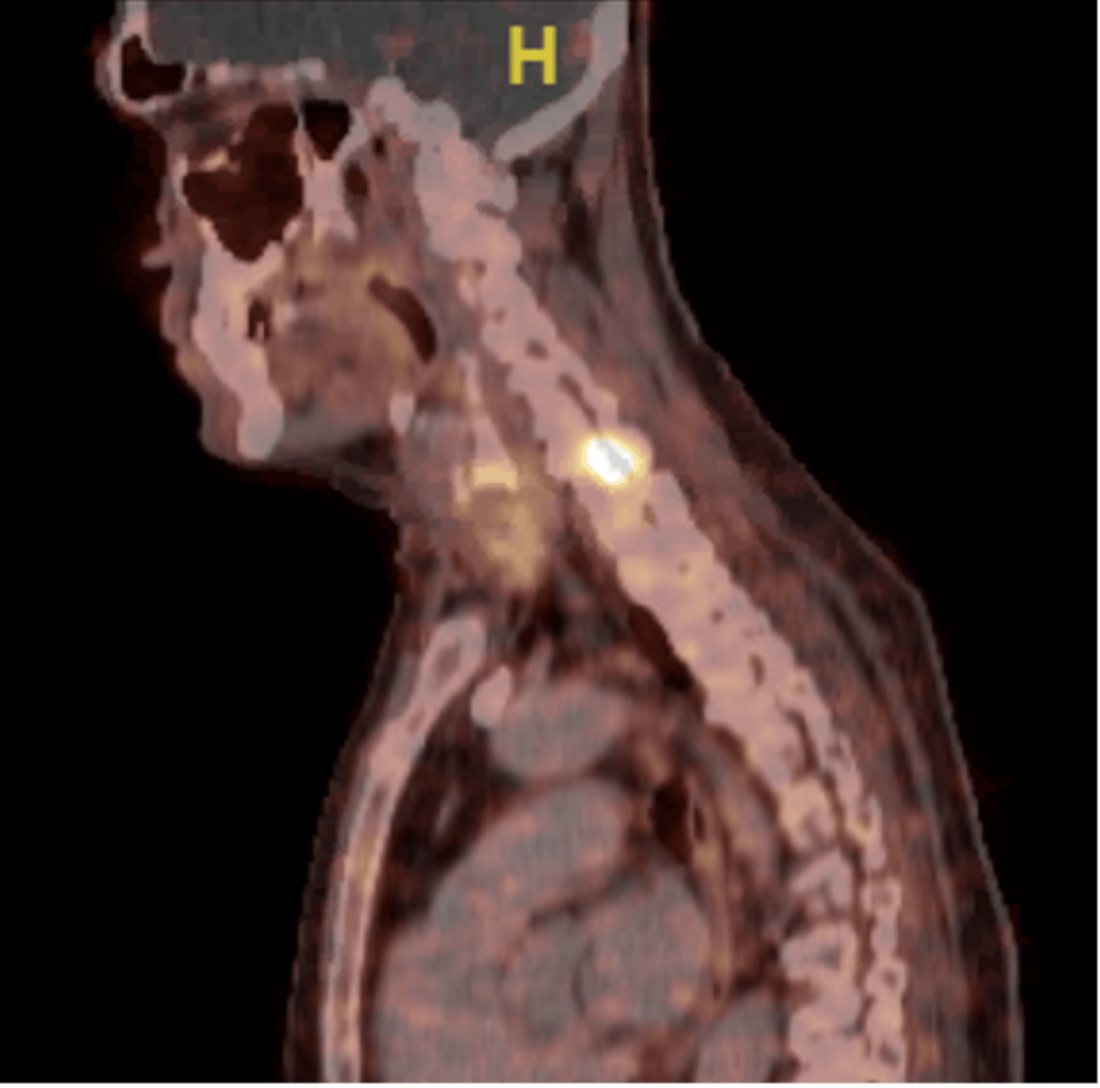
Hyperdense lesion in C7-T1 concerning for a mesenchymal tumor Radiographic imaging via 68Ga-DOTATATE-based positron emission tomography (PET)/computed tomography (CT) revealed a hyperdense lesion in C7-T1 concerning for a mesenchymal tumor.
